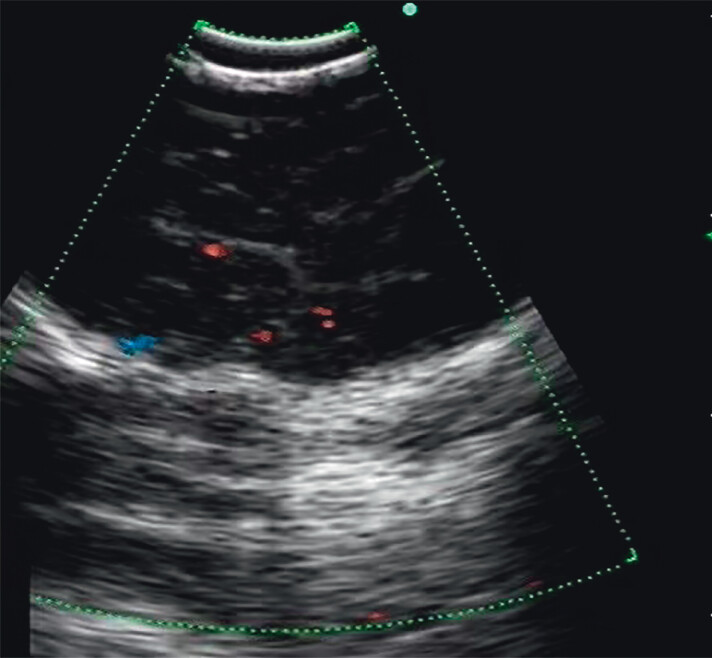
Endoscopic ultrasound revealed a hypoechoic area in the left aryepiglottic fold, with well-defined boundaries and low internal vascularity.
